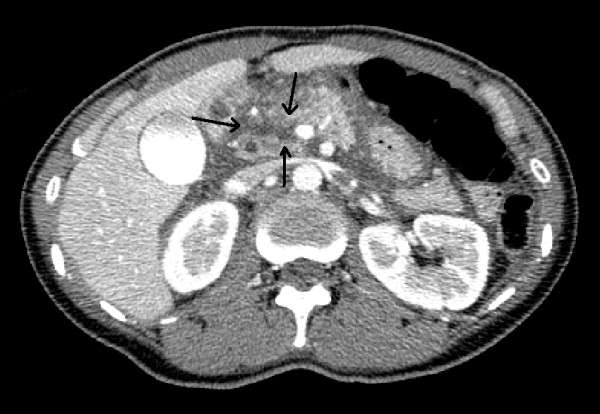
A computed tomography scan showed pancreatic parenchyma disruption with a small amount of peripancreatic fluid at the pancreatic head.
